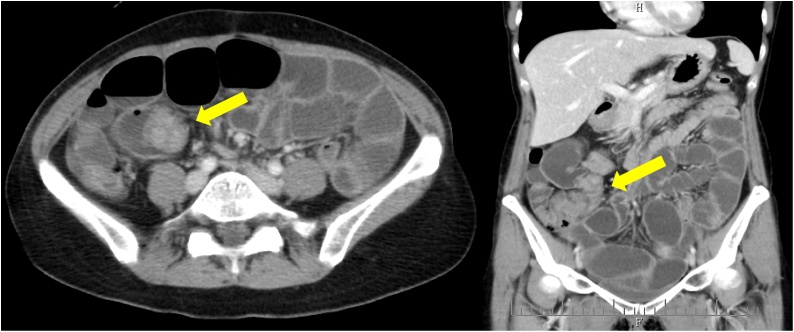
Computed tomography findings. A tumor was observed at the end of the ileum and the proximal intestinal tract was expanded, which suggested obstructive ileus (arrow).
